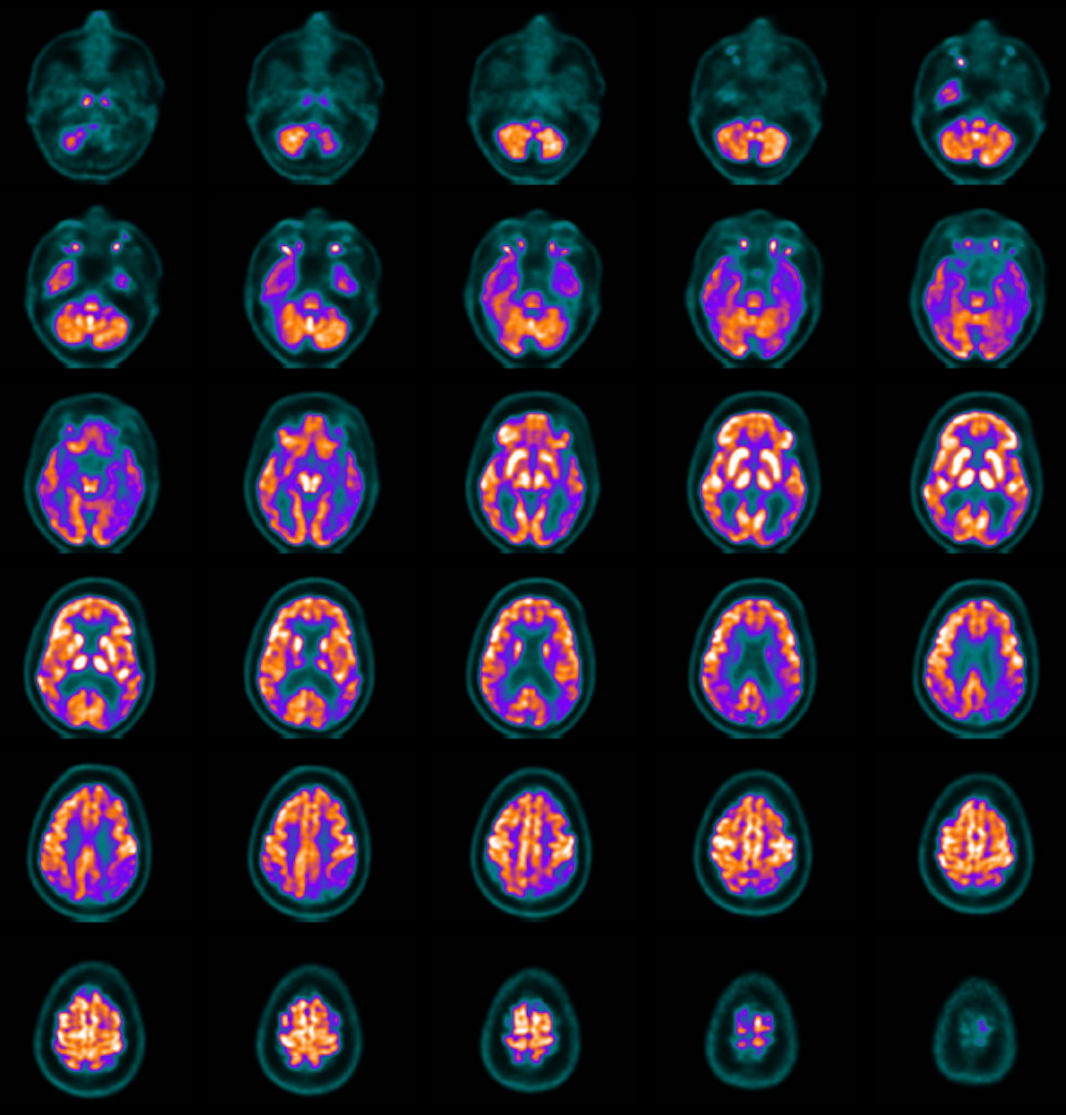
FDG-PET of a patient with Alzheimer's disease. Transversal slices show
